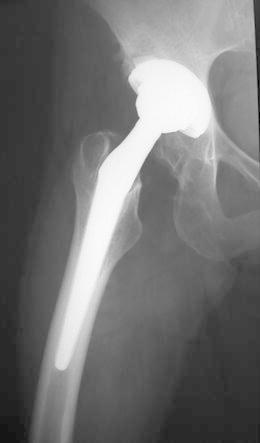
Radiographic aspect in axial view
